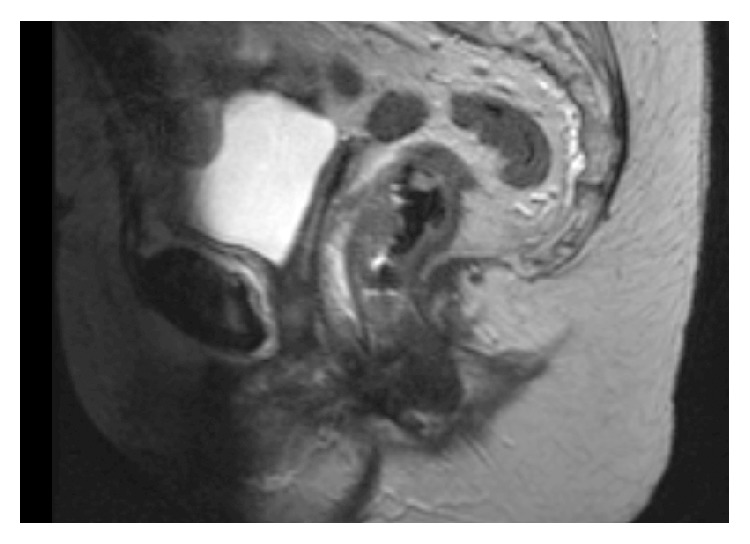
Staging MRI demonstrating low rectal mass, invading potential anterior resection margin.
